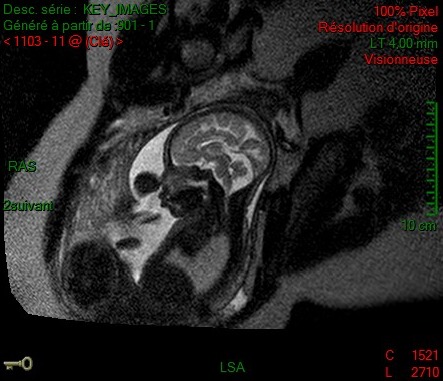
Sagittal MRI performed at 32 WG. Short corpus callosum
